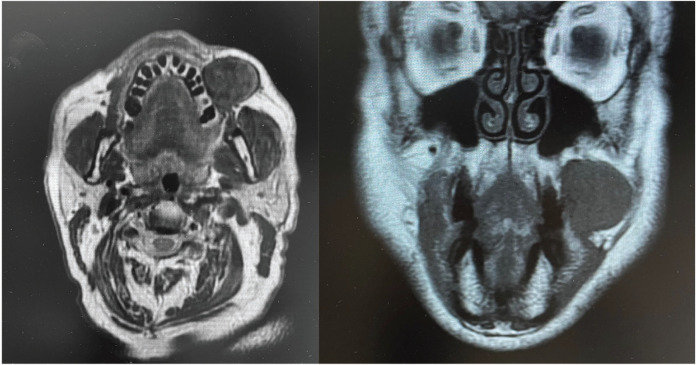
MRI images showing a well-defined mass in the buccal space.
